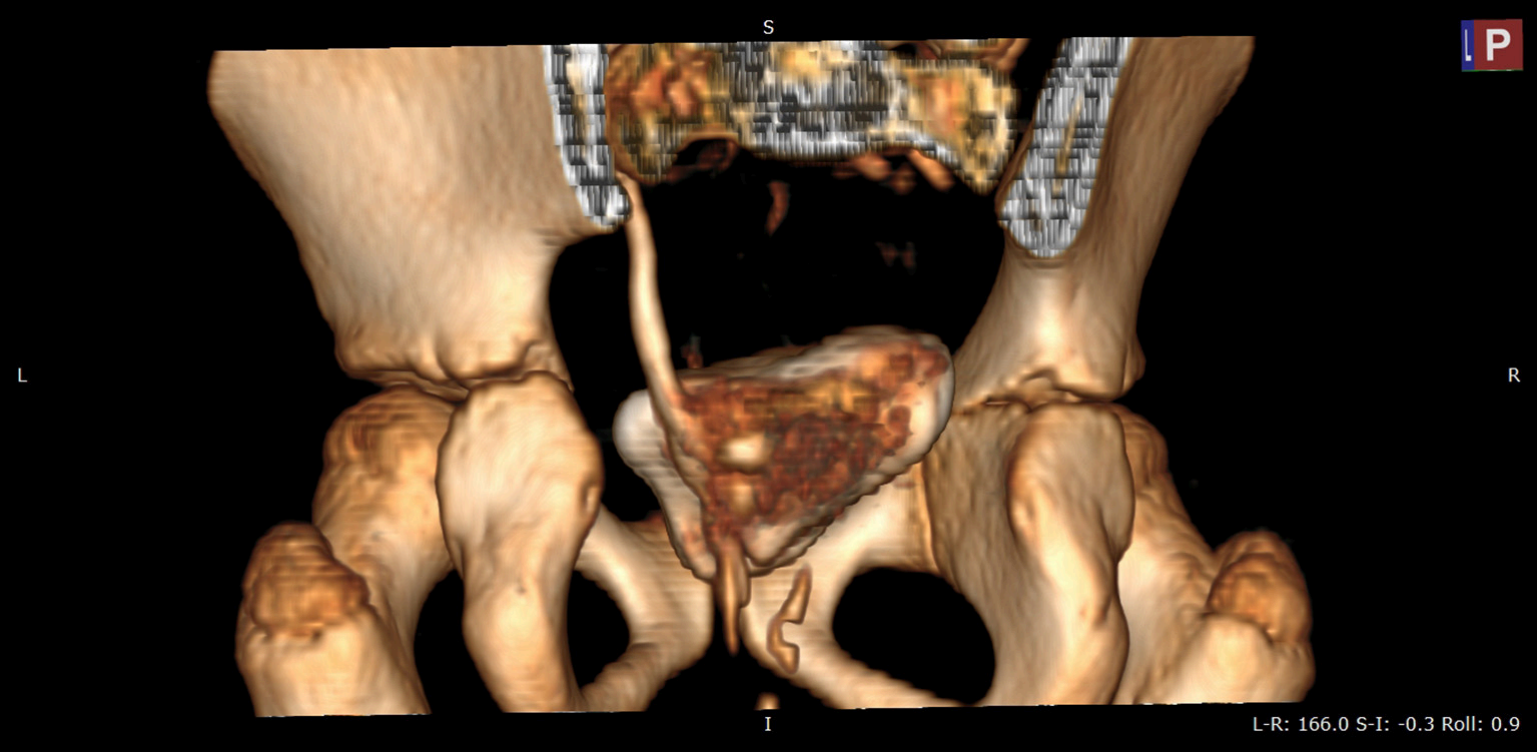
VRT (volume rendering technique) CT image, urographic sequence—posterior view after removing sacral bone—paravesical path of left ureters before insertion into vagina.
